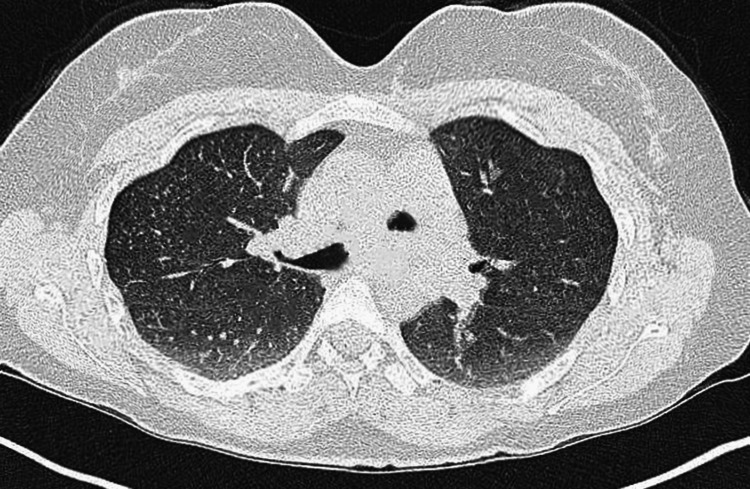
Diffuse centrilobular ground-glass nodules along the bronchovascular structures in chest CT.
